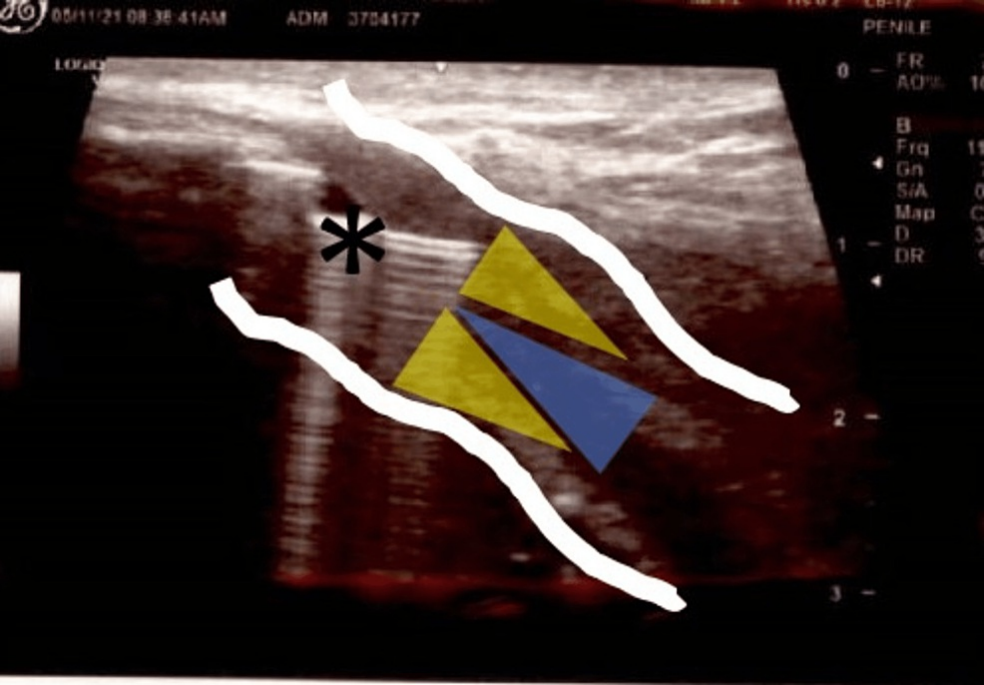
Intraoperative urethral ultrasound Asterisk = cystoscopic injection needle in the dorsal spongy tissue with shadowing. White lines = tunica albuginea. Yellow shaded area = spongiofibrosis. Blue shaded area = urethral lumen.
